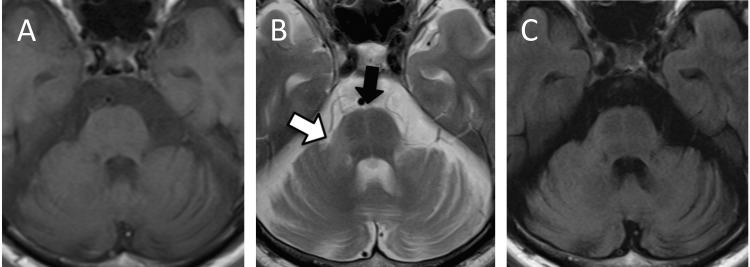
Brain MRI findings Brain MRI shows atrophy of cerebellar and middle cerebellar peduncles (MCP), enlargement of the fourth ventricle, hyperintensity in the MCP (MCP-sign) (white arrow), and hot cross bun sign (black arrow). A: Axial T1-weighted MRI, B: Axial T2-weighted MRI, C: Fluid attenuated inversion recovery (FLAIR)
